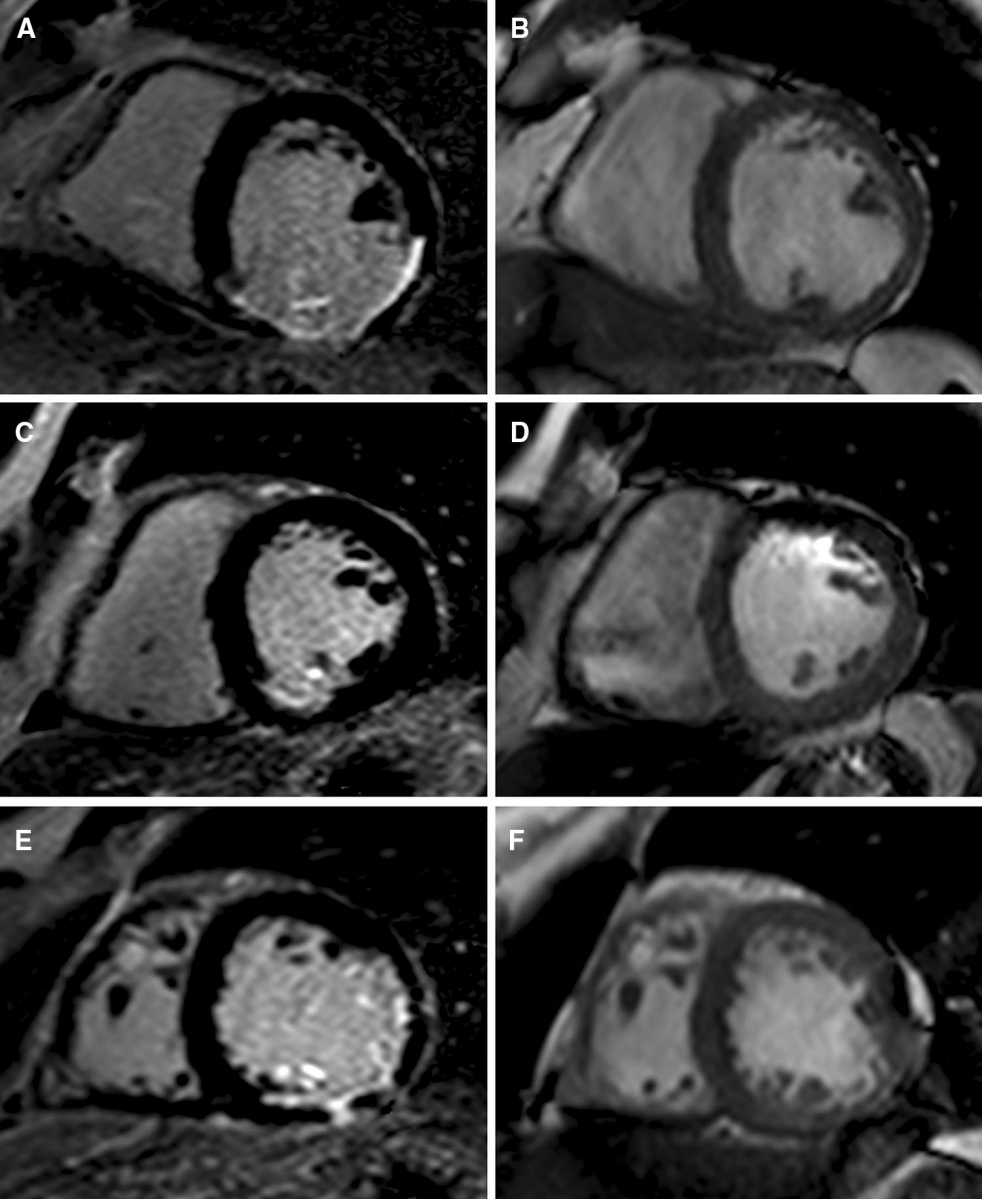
Assessment of PMI by LGE cardiac MRI. Patient with inferolateral STEMI with complete infarction of the posteromedian PM. A LGE image. B Cine image of the same location as a side-by-side reference for localizing the PM within the blood pool during interpretation of contrast-enhanced images. Patient with inferior STEMI and partial infarction of the posteromedian PM. C LGE image. D Cine image. Patient with anterolateral and inferior STEMI and combined partial infarction of the anterolateral PM and complete infarction of the posteromedian PM. E LGE image. F Cine image. *(AL)PM* (anterolateral) papillary muscle, *LGE* late gadolinium-enhancement, *MRI* magnetic resonance imaging, *PMI* papillary muscle infarction, *(PM)PM* posteromedian papillary muscle, *STEMI* ST elevation myocardial infarction
